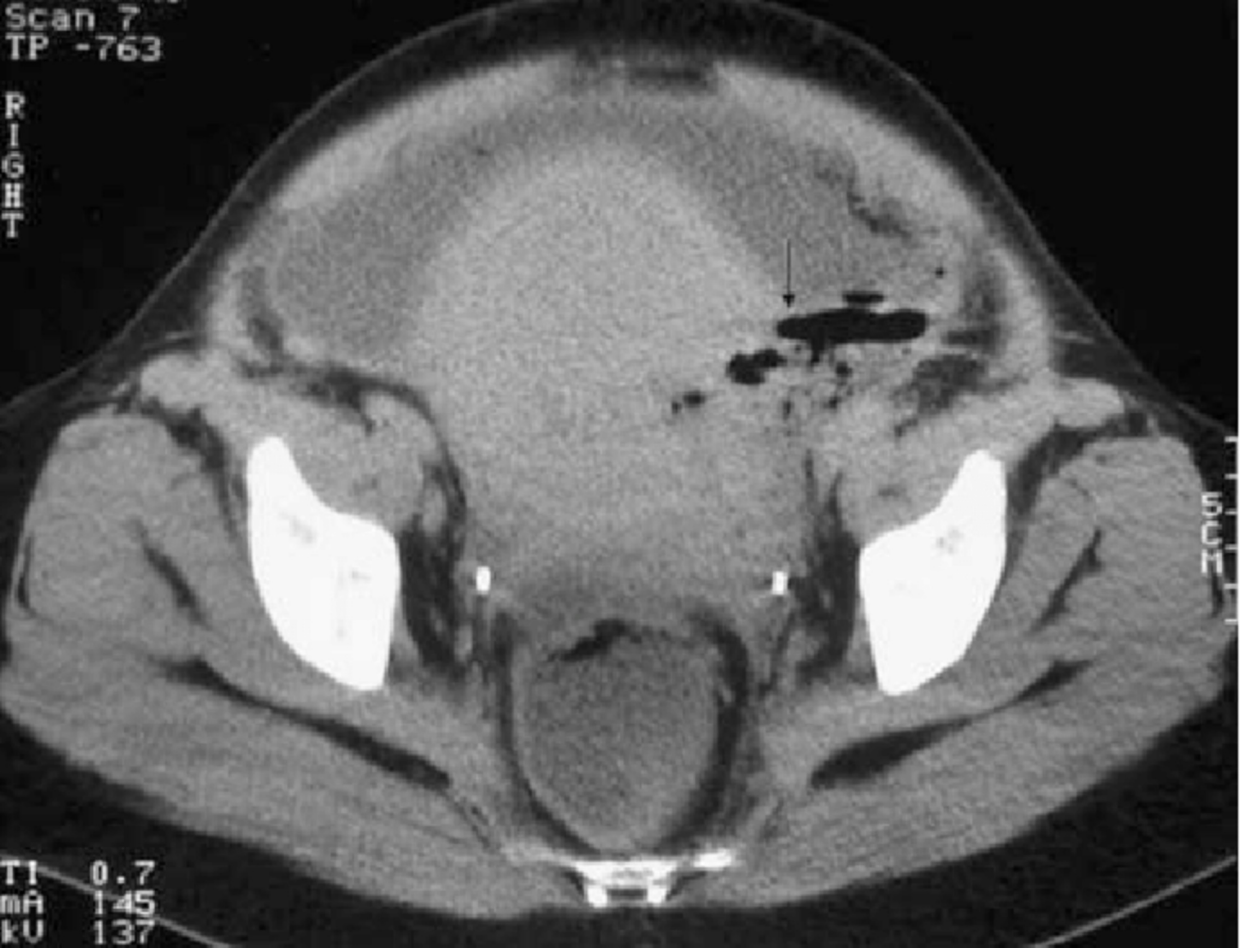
CT showing air bubbles spreading through the ruptured uterus into the abdominal cavity
